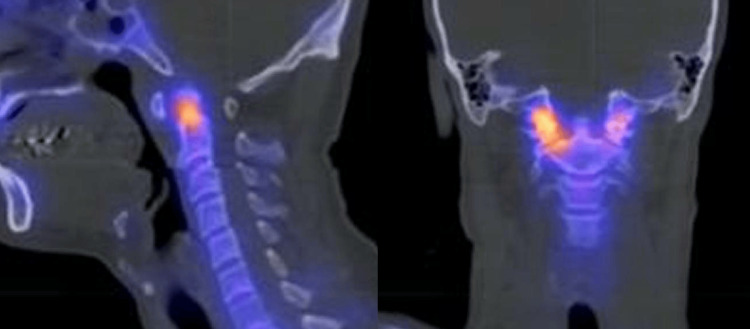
SPECT images revealed atlanto-axial arthrosis with active C1-C2 and occipital diastasis SPECT: single-photon emission computed tomography
